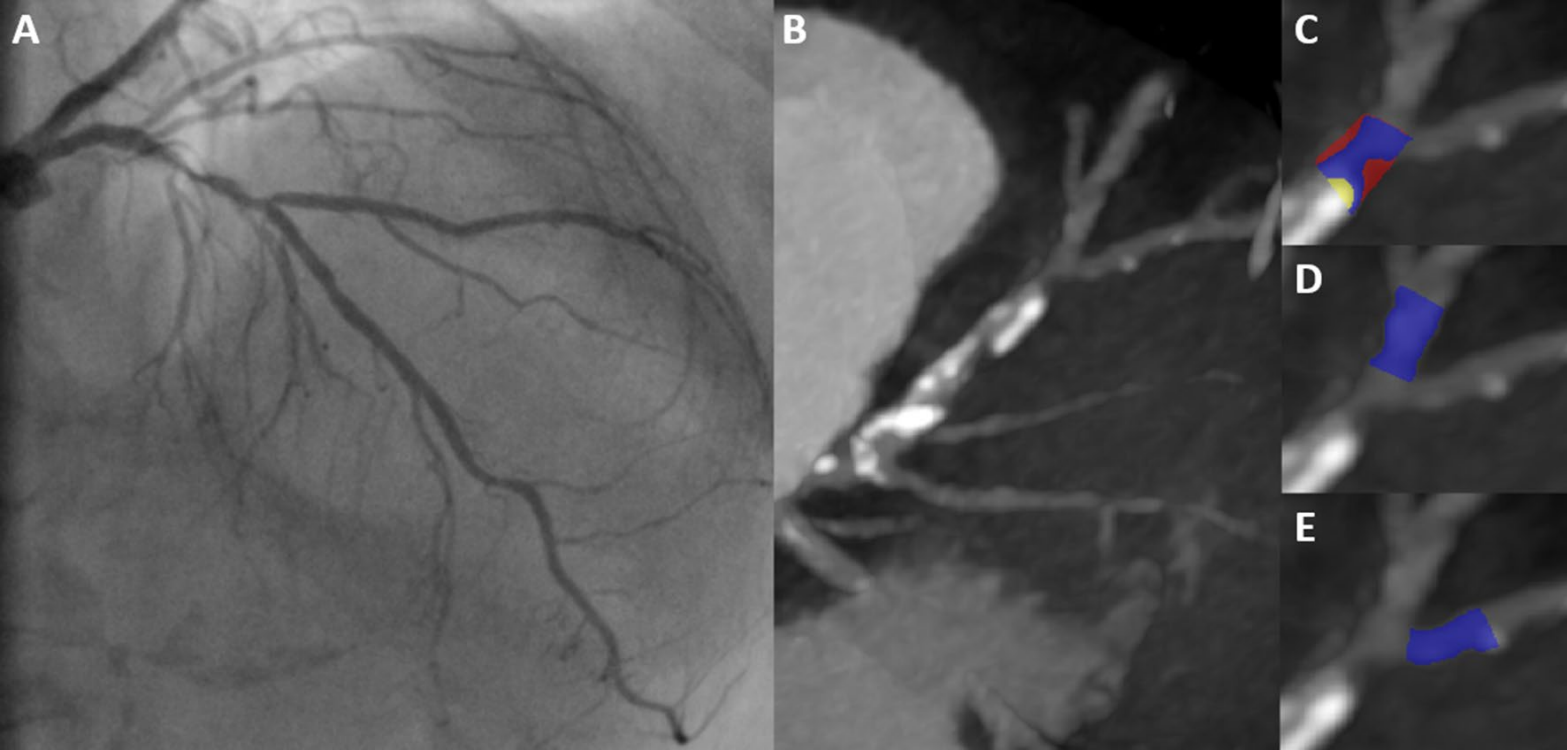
An example of the coronary bifurcation lesion in invasive angiography (a) and its corresponding image in computed tomography angiography (b). Plaque was quantitatively analyzed in the respective bifurcation segments: proximal main vessel (c), distal main vessel (d) and side branch (e). Blue represents lumen, yellow—calcified plaque, and red—noncalcified plaque. In the above lesion, coronary plaque—defined as tissue greater than 1 mm^2^ associated with the coronary wall—was present only in the proximal segment of the main vessel
